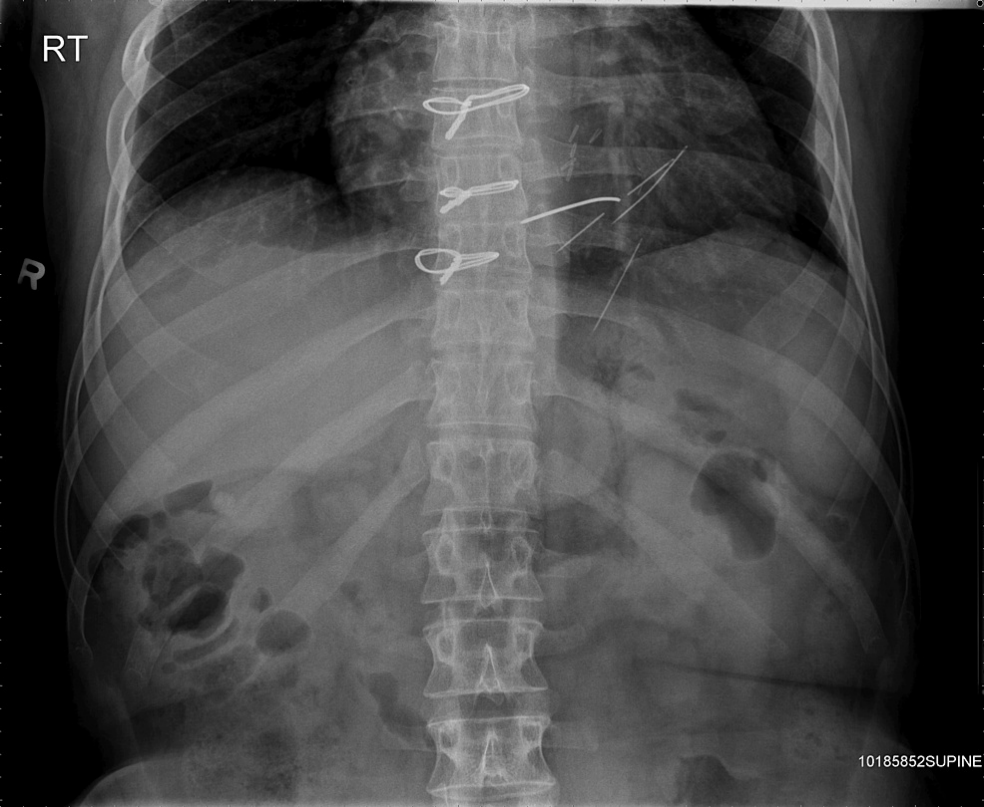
X-ray with a paperclip and sewing needle inserted in the pericardium and myocardium.
